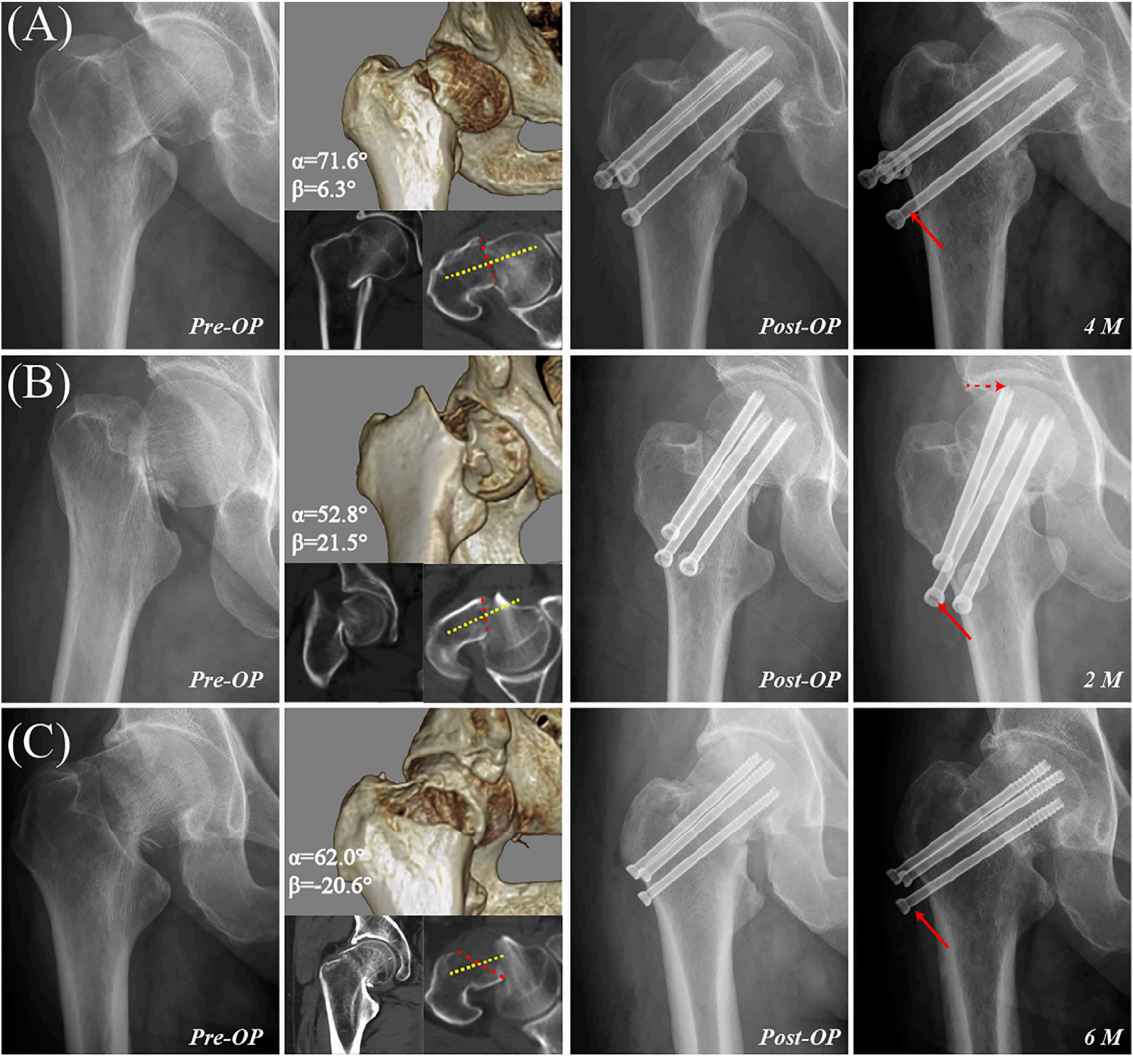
Examples of femoral neck fractures with 3-D unstable inclination angle. (A) A 46-year-old male with a vertical unstable inclination angle (α = 71.6° and β = 6.3°) was treated with three cannulated screws. Radiography taken 4 months postoperatively revealed severe screw withdrawal (red arrow) as well as femoral neck shortening, varus collapse, and delayed union. (B) A 46-year-old female with retroversion oblique unstable inclination angle (α = 52.8° and β = 21.5°) was treated by three cannulated screws with a fair reduction quality. Radiography taken 2 months postoperatively revealed severe screw withdrawal (solid red arrow) as well as femoral neck shortening, varus collapse, screw penetration of the joint capsule (dotted red arrow), and delayed union. (C) A 59-year-old male with anteversion oblique unstable inclination angle (α = 62.0° and β = −20.6°) was treated with three cannulated screws. Radiography taken 6 months postoperatively revealed severe screw withdrawal (red arrow) as well as femoral neck shortening, varus collapse, and delayed union.
